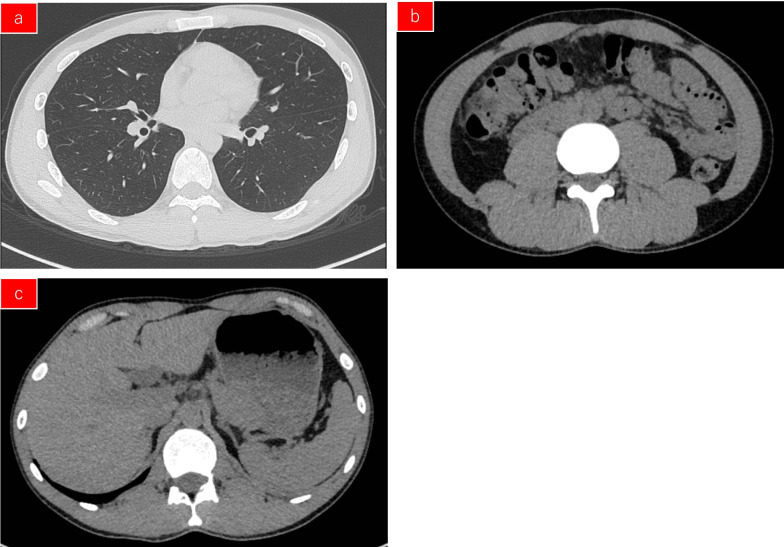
Computed tomography revealed no pneumonia (a), intestinal oedema (b), hepatomegaly (c), or lymphadenopathy (a-c)
